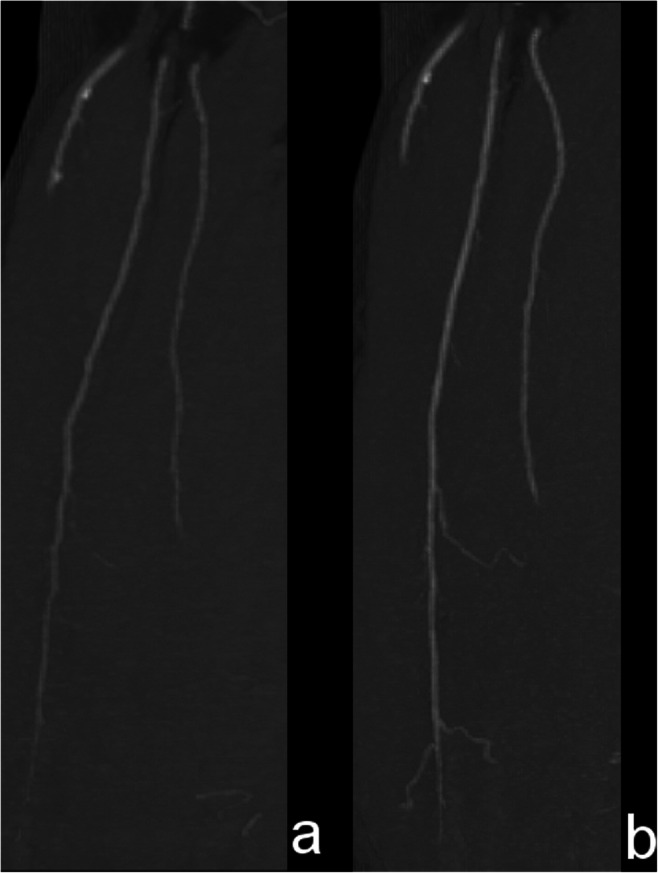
CTA angiograms – lower leg longitudinal projection, shows the increase in length of collaterals; a: before plasmid administration; b: after 3 months from plasmid administration
